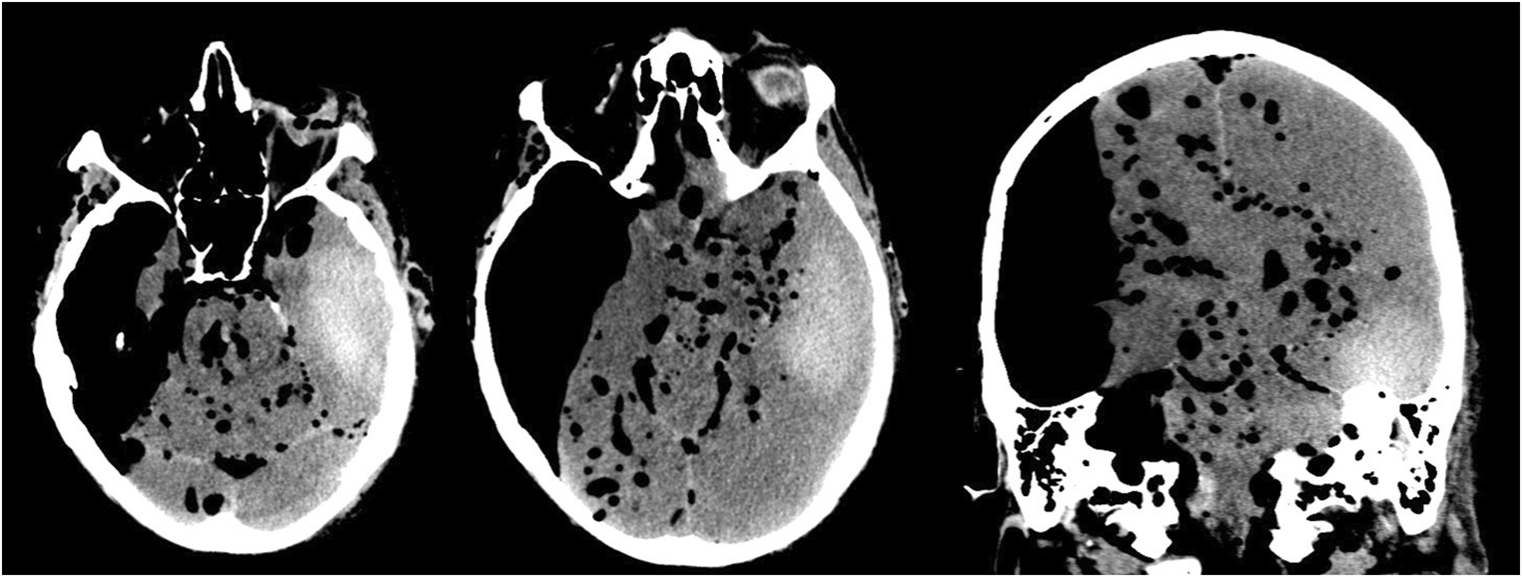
Temporal bleeding shown by CT
